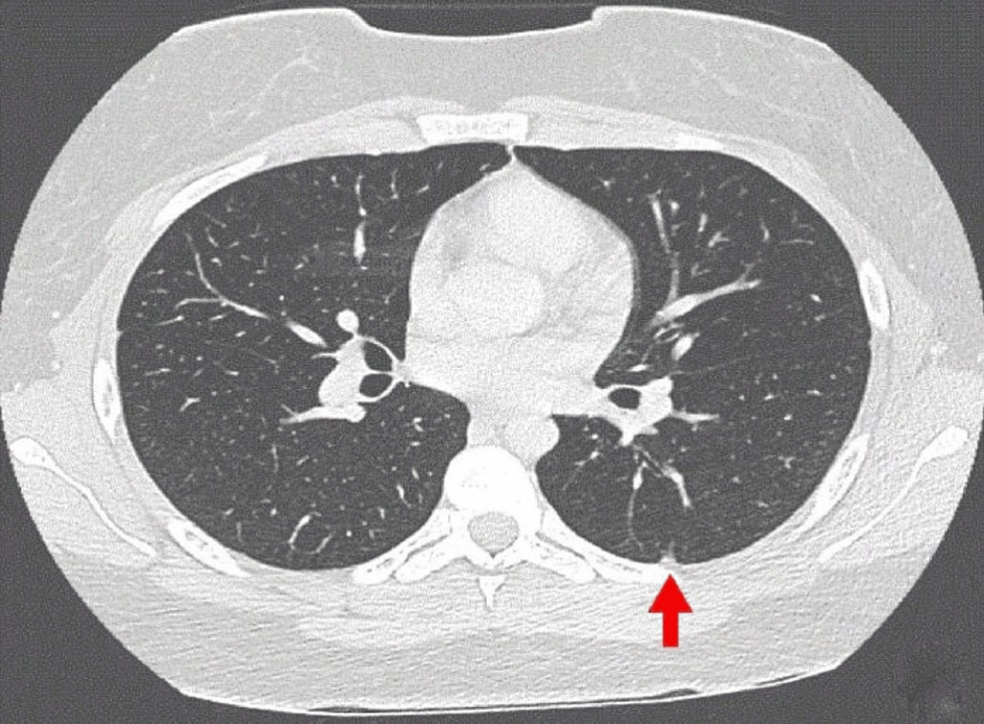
Chest computed tomography of Case 2 showing benign-looking subpleural nodules (arrow).
